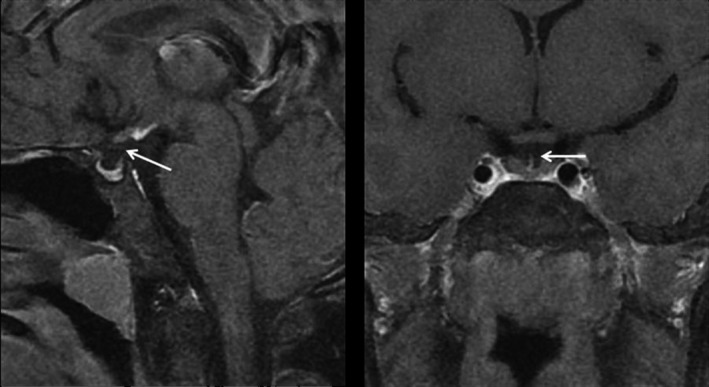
The sagittal and coronal pituitary on MRI. Left panel: The sagittal image showing the ectopic pituitary located at the floor of the third ventricle, along with a small anterior pituitary gland. Right panel: The coronal image showing the absence of pituitary stalk.
